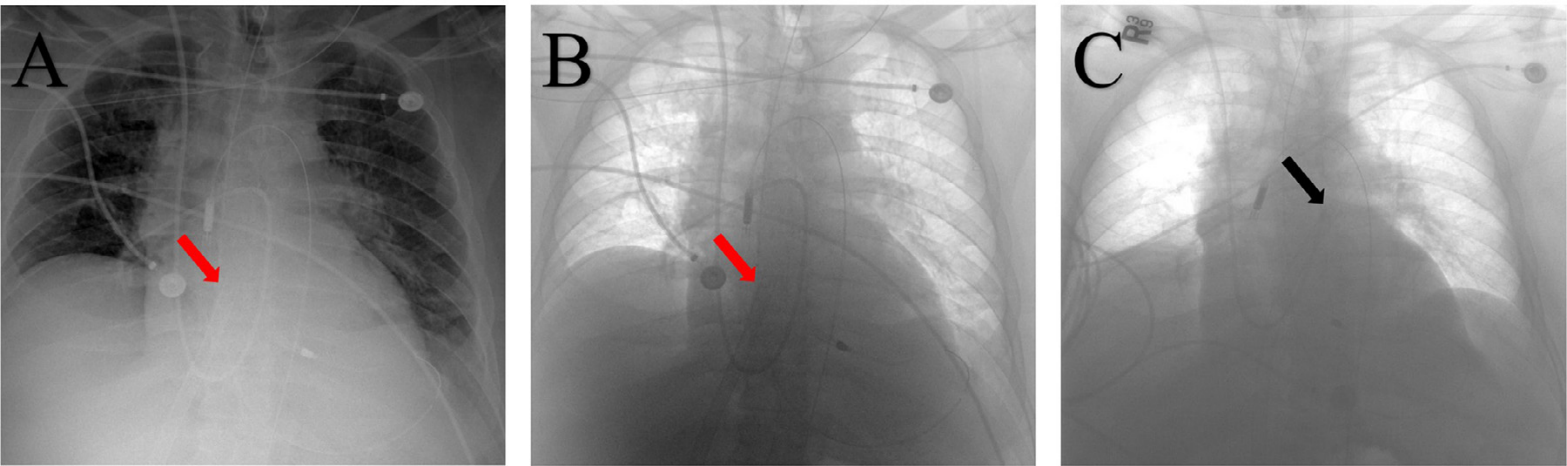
(A, B) Chest radiograph (normal and inverted) confirming the extracorporeal membrane oxygenation venous canula position shows slight migration from the superior vena cava–right atrium junction to the middle of the right atrium. (C) Inverted chest radiograph shows the migration of the venous cannula to the left atrium. Arrows indicate the tip of the extracorporeal membrane oxygenation venous cannula. The pulmonary artery catheter shown is deep, and the position was adjusted.
